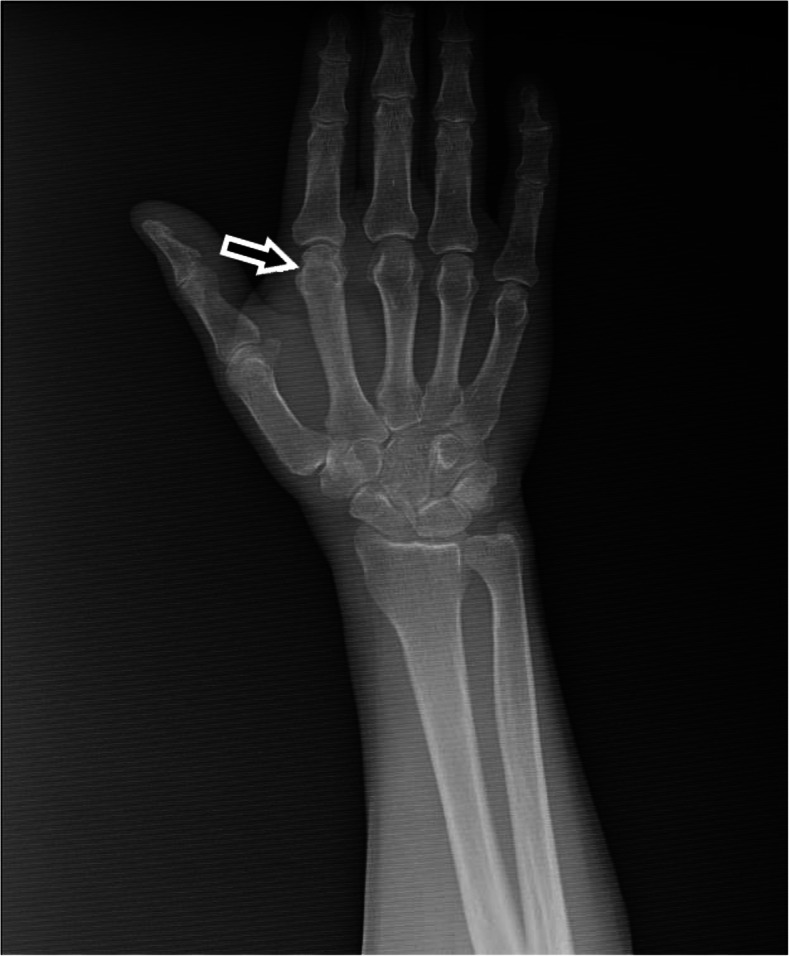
Conventional radiograph of the right hand and second MCP joint of our patient. The arrow shows the suspected erosion in the second MCP joint
